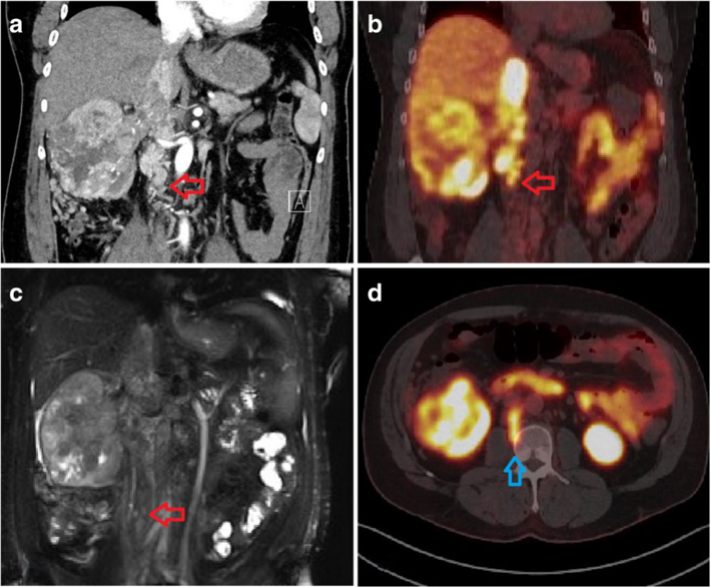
Comparison of CT, MRI and PSMA PET in subject 1. a Contrast CT demonstrates large renal lesion with IVC thrombus extending down to bilateral lower limbs. b PSMA PET demonstrates avidity within primary tumour and tumour thrombus down to the level of bland thrombus (*red arrow*). c MRI showing tumour within IVC, surrounded by bland thrombus. d Axial PSMA PET demonstrates tumour thrombus extending into the lumbar vein, which was not identified by other imaging modalities—*blue arrow*
